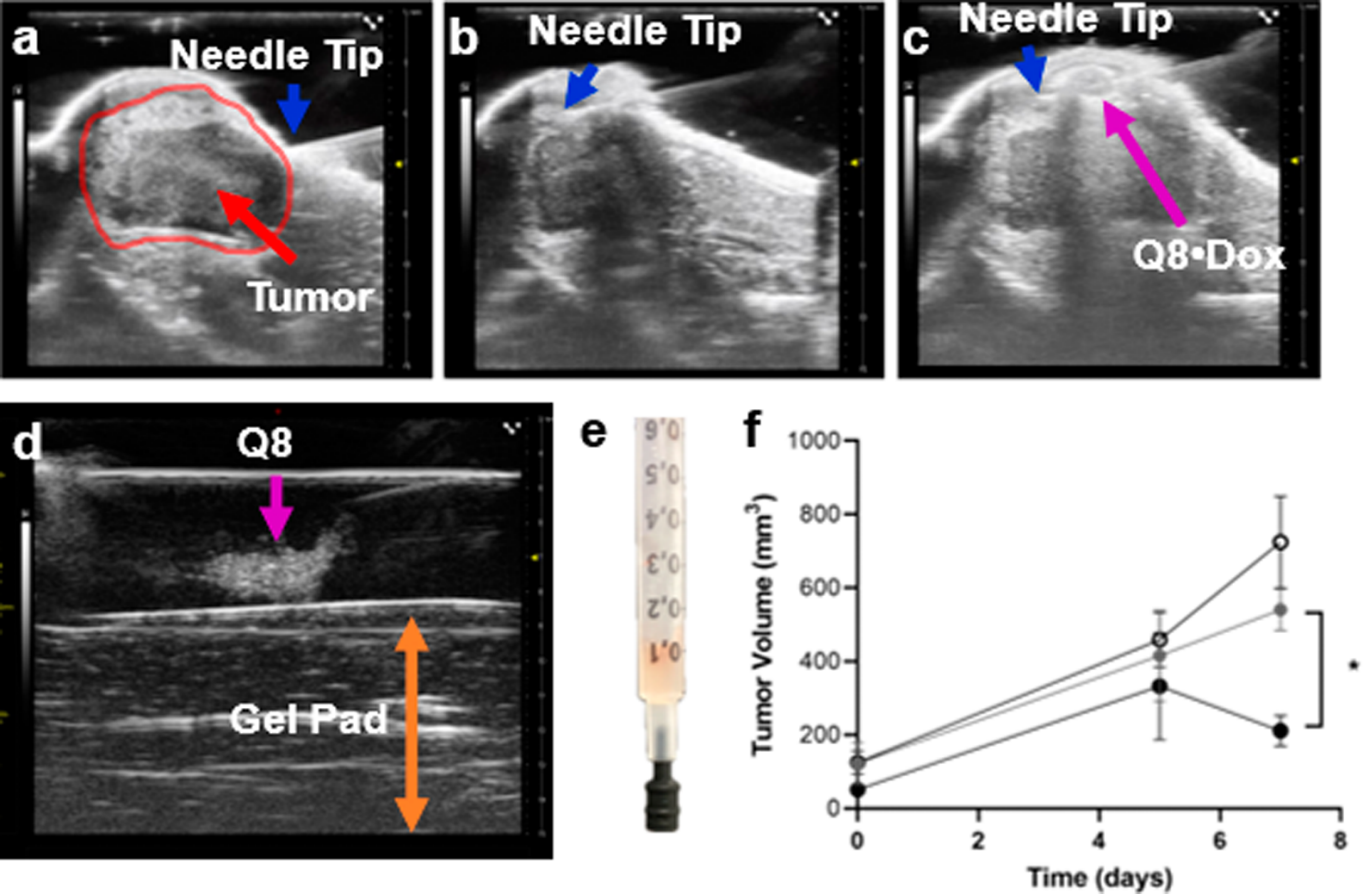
Ultrasound-guided injection. (a) Axial ultrasound view
